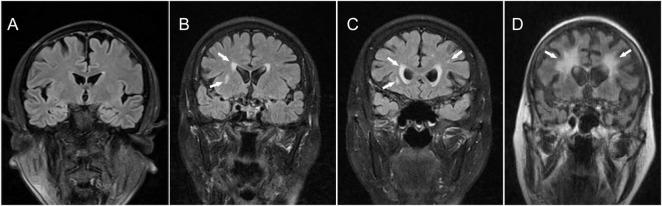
Magnetic resonance imaging examination of the brain. (A) Grade-0 represents normal or non-dots WMHs; (B) Grade-1 presents multiple dots WMHs; (C) Grade-2 is a fusion lesion with punctured WMHs junctions, which can also be found in deep white matter; (D) Grade-3 showed extensive fusion of WMHs with brain atrophy. White arrows represent WMHs.
